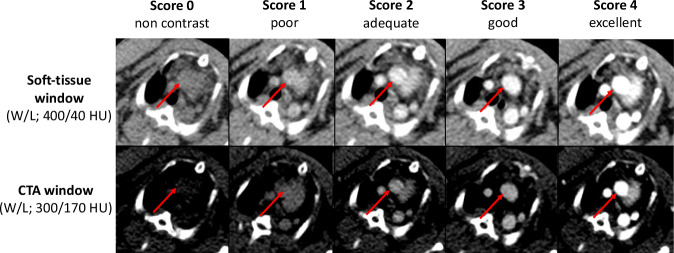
Representative vascular conspicuity scores demonstrated at the upper mediastinal level. Vascular conspicuity scores were demonstrated by representative axial images at the upper mediastinal level. Vascular conspicuity is subjectively graded as non-contrast, poor, adequate, good, and excellent (from left to right); corresponding images are demonstrated at soft tissue (top row; width/level (W/L) = 400/40 HU) and CTA window levels (bottom row; W/L; 300/170 HU). The ascending aorta is indicated with a red arrow. CTA, Computed tomography angiography; L, Level; W, Width
